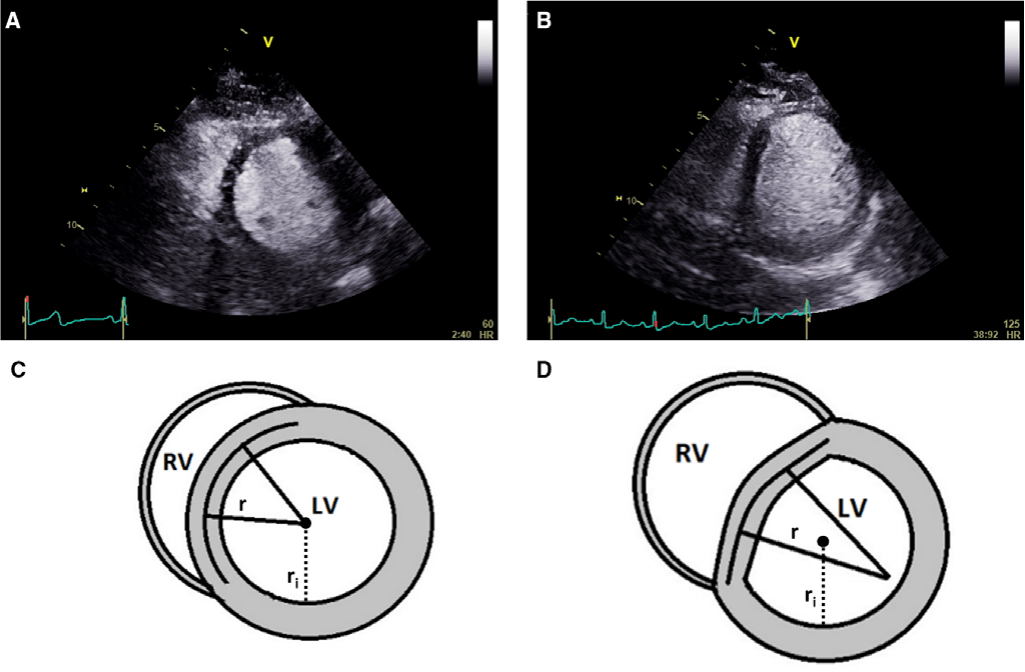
Septal flattening from diastolic ventricular interaction causes a D‐shaped left ventricle, and can be quantified from the normalized radius of curvature. A, A resting contrast echocardiogram in the parasternal short axis view of the left ventricle of a patient with heart failure with preserved ejection fraction, demonstrating a circular interventricular septum at end‐diastole. B, In the same patient during exercise, the subsequent development of pulmonary hypertension leads to pericardial constraint and diastolic ventricular interaction, flattening the septum and resulting in a D‐shaped LV. C, The shape of the left ventricular short axis image is calculated from the ratio of the radius extrapolated from the demarcated septum (*r*) to the cavity radius (*r*
